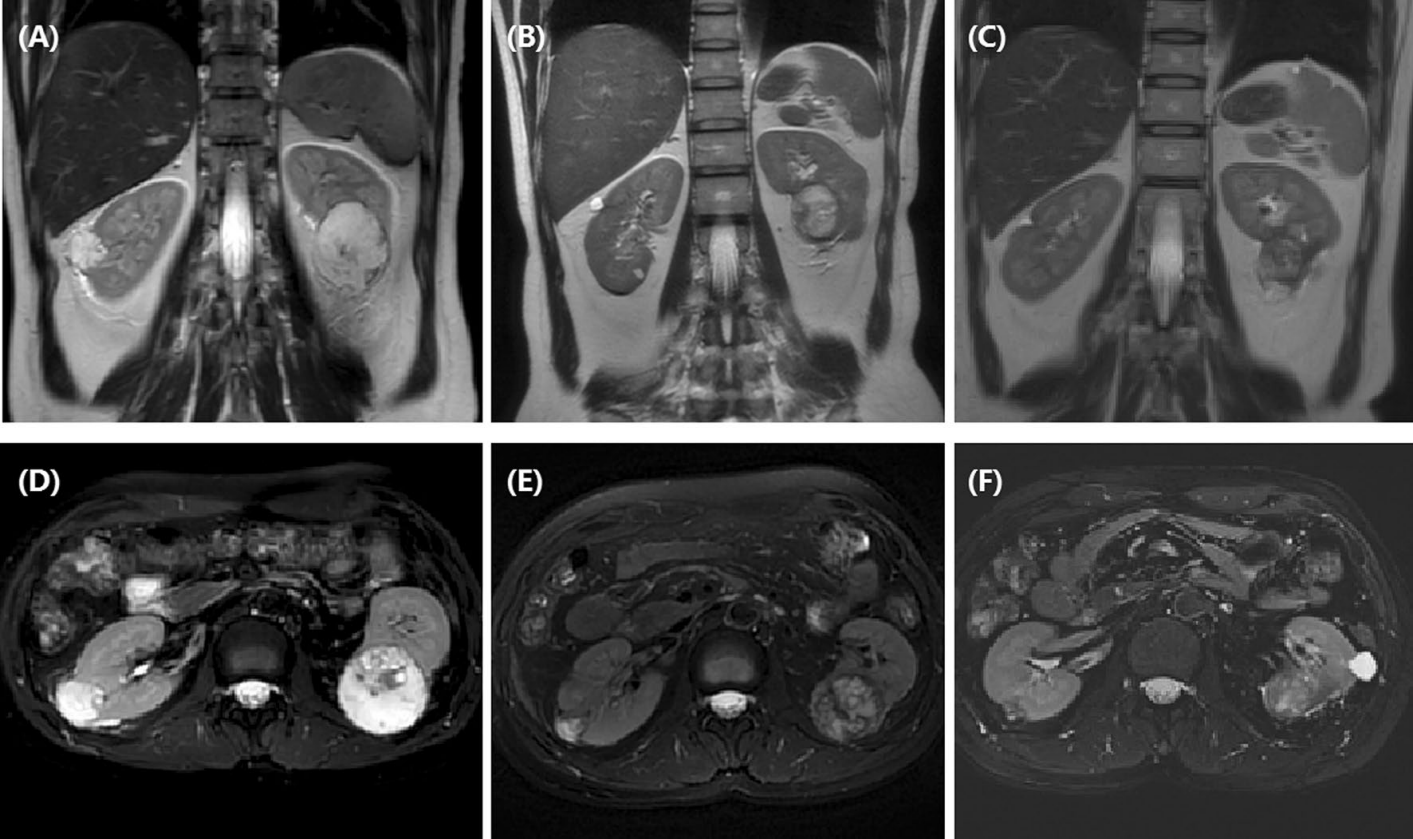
A 37-year female with bilateral RCCs followed up by MRI. (A) T2W-TSE series in coronal view showed bilateral RCCs before treatment. (B) Tumors shrunk significantly 1 month after DEB-TACE. (C) The right RCC almost disappeared after 3 months. T2-SPIR series in cross-sectional view showed the change of bilateral RCCs before (D), 1 month (E) and 3 months later (F).
